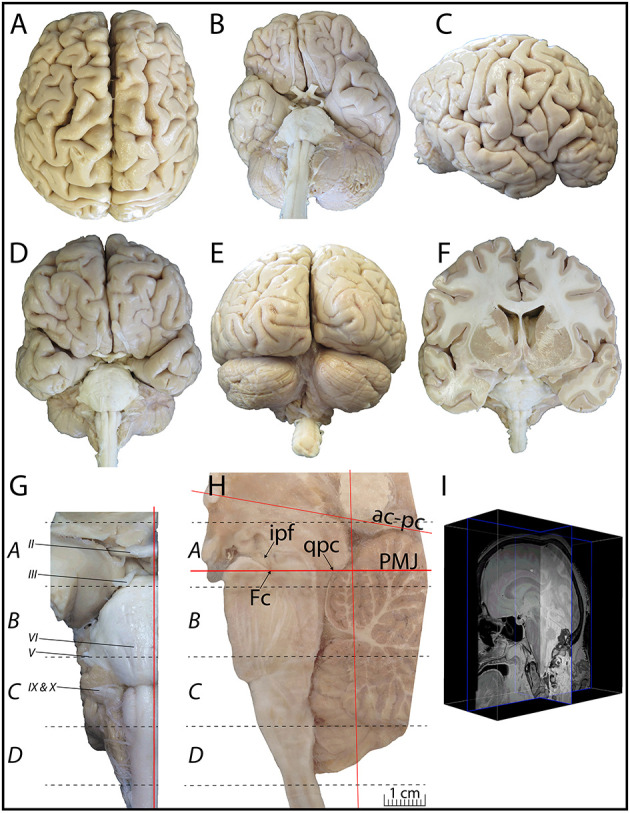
(A–E) Superior (A), inferior (B), lateral (C), anterior (D), and posterior (E) views of the whole brain used. This brain comes from a generous donation of a 61-year-old woman who died from a pluri-metastatic colorectal cancer with no signs of neurological, neurodegenerative or psychiatric diseases. The perfused and extracted brain weighed 1,328 g. (F) Coronal view of the sectioned brain. (G) Picture of the dissected brainstem cut through its longitudinal axis, along a sagittal plane, at 1 mm from the midline. (H) From the right hemi-brainstem containing the midline, four blocks were cut along the ponto-mesencephalic junction plane (PMJ in H). Block A extends from 11.19 to −0.98 mm (plates 1–11), block B from −3.87 to −21.33 mm (plates 12–24), block C from −24.09 to −36.87 mm (plates 25–38), and block D from −39.70 to −51.70 mm (plates 39–45), relative to the PMJ. (I) Brain MRI is available as Supplementary Material.
